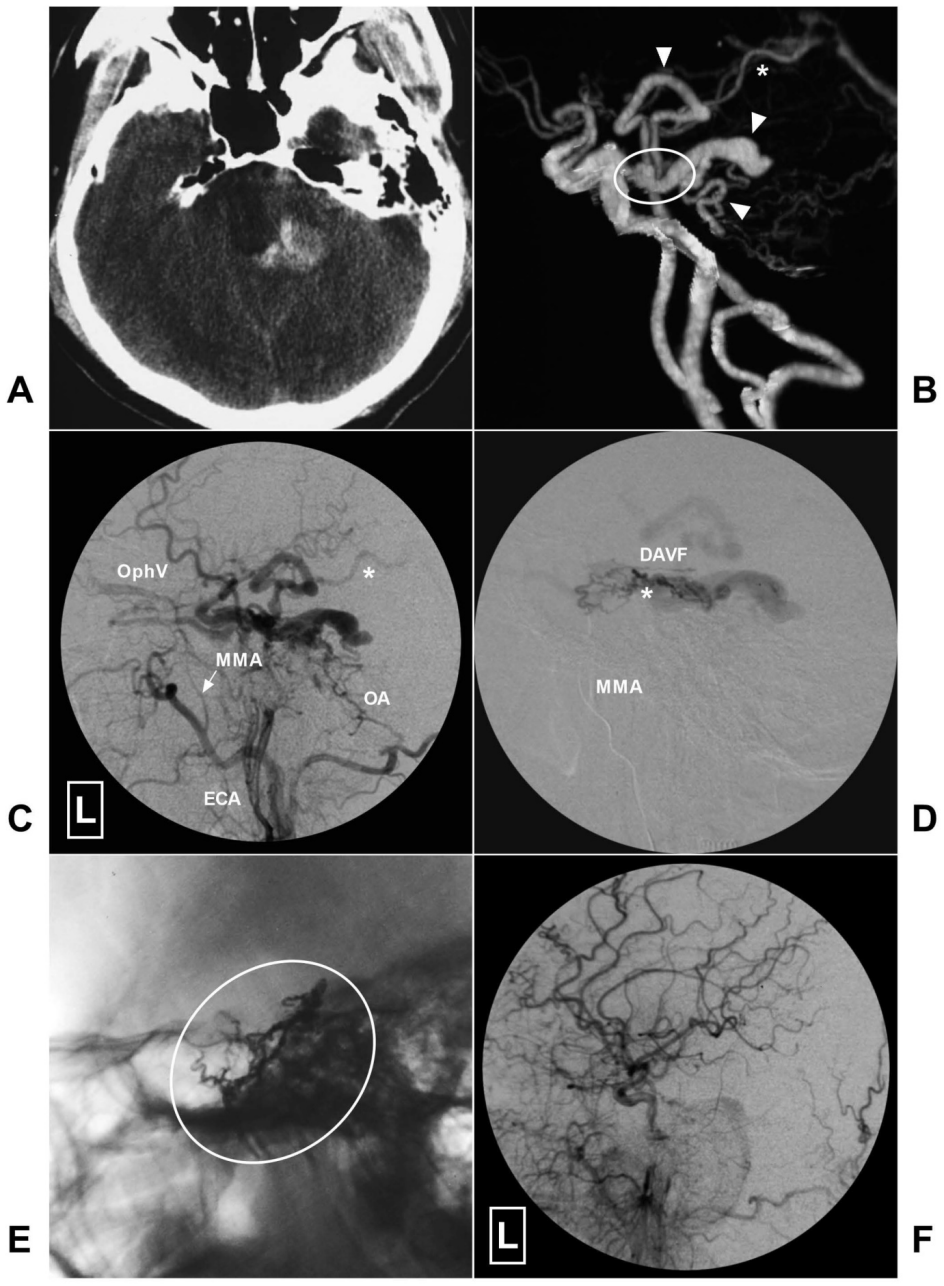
A, CT shows hemorrhage at the left side posterior to the brainstem. B, CTA reveals dilated venous structure (arrow heads) around the brainstem draining to the Vein of Galen (asterisk) indicating a petroclival DAVF (encircled area). C, Angiogram of the left ECA in lateral view shows the DAVF is mainly fed by the MMA (arrow) and OA and drains to the peri-brainstem and ophthalmic vein. Asterisk denotes the vein drains to the Vein of Galen. D, Super-selective angiogram via microcatheter shows the DAVF. Asterisk denotes the fistula point. E, X-ray of the cranium reveals Onyx casting (encircled area) through the MMA. F, Angiogram of the left CCA in lateral view after EVT shows the DAVF is completely obliterated. Abbreviations: CCA, common carotid artery; CT, computed tomography; CTA, computed tomography angiography; DAVF, dural arteriovenous fistula; ECA, external carotid artery; L, left; MMA, middle meningeal artery; OA, occipital artery; OphV, ophthalmic vein.
